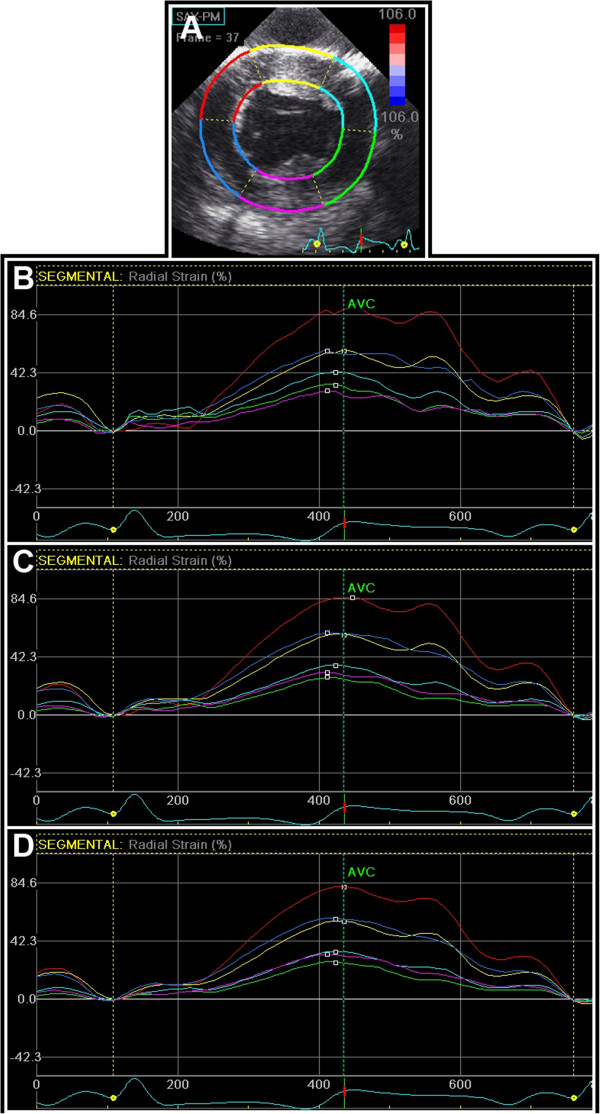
Radial strain curves with different degrees of user controlled spatial and temporal smoothing. (A) Epicardial left ventricular short-axis view used for 2-dimensional strain analysis with Speckle Tracking Echocardiography. The left ventricle is divided into 6 standard segments, labeled AntSept (yellow), Ant (cyan), Lat (green), Post (pink), Inf (blue) and Sept (red). Regional radial and circumferential strain was measured within each segment. The anterior and posterior wall was defined as the AntSept and Post segment, respectively. Global strain was calculated as the average value of the six segmental strain values at each frame throughout the heart cycle. The curves show radial strain at baseline with (B) minimal, (C) factory default and (D) maximal degrees of both spatial and temporal smoothing.
